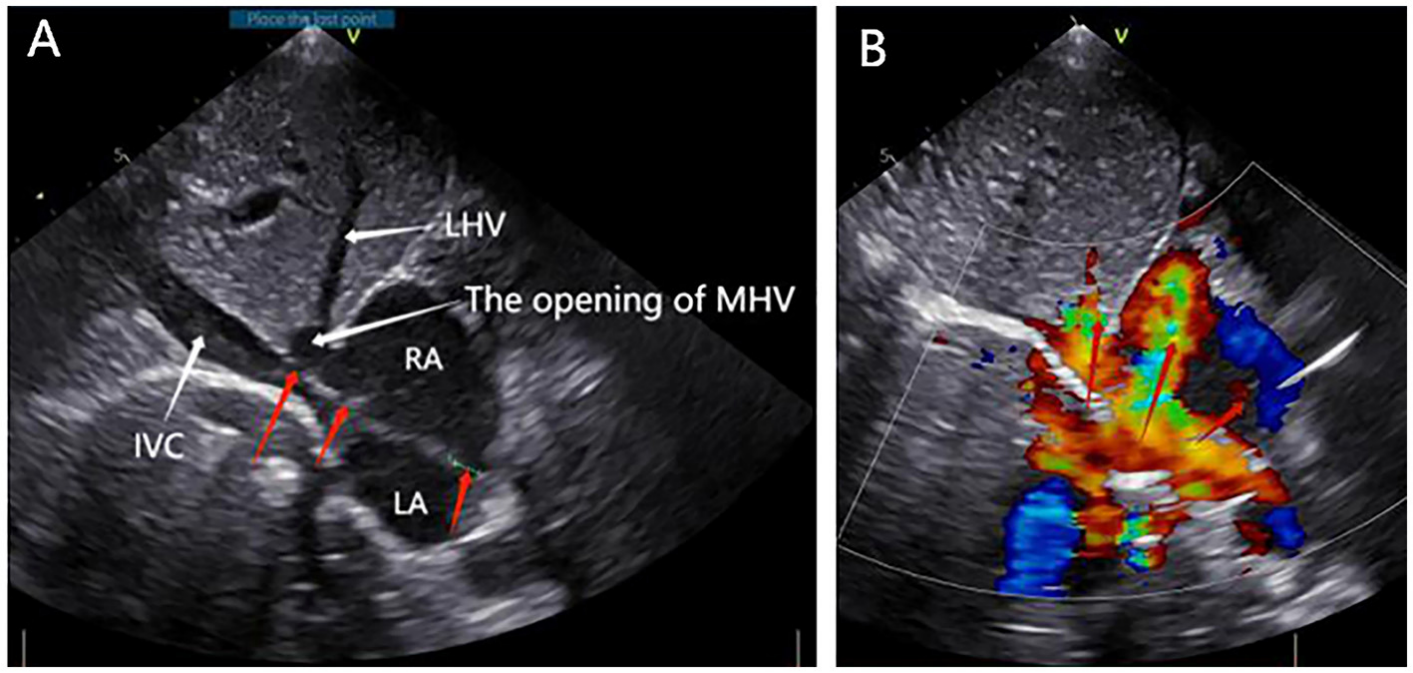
Preoperative transthoracic echocardiography. (A) Right atrium (RA), left atrium (LA), left hepatic vein (LHV), the opening of middle hepatic vein (MHV), inferior vena cava (IVC) (white arrowheads), and three atrial septal defects (ASD) (red arrowheads). (B) Blood flowed from the left atrium to the right atrium (red arrowheads).
